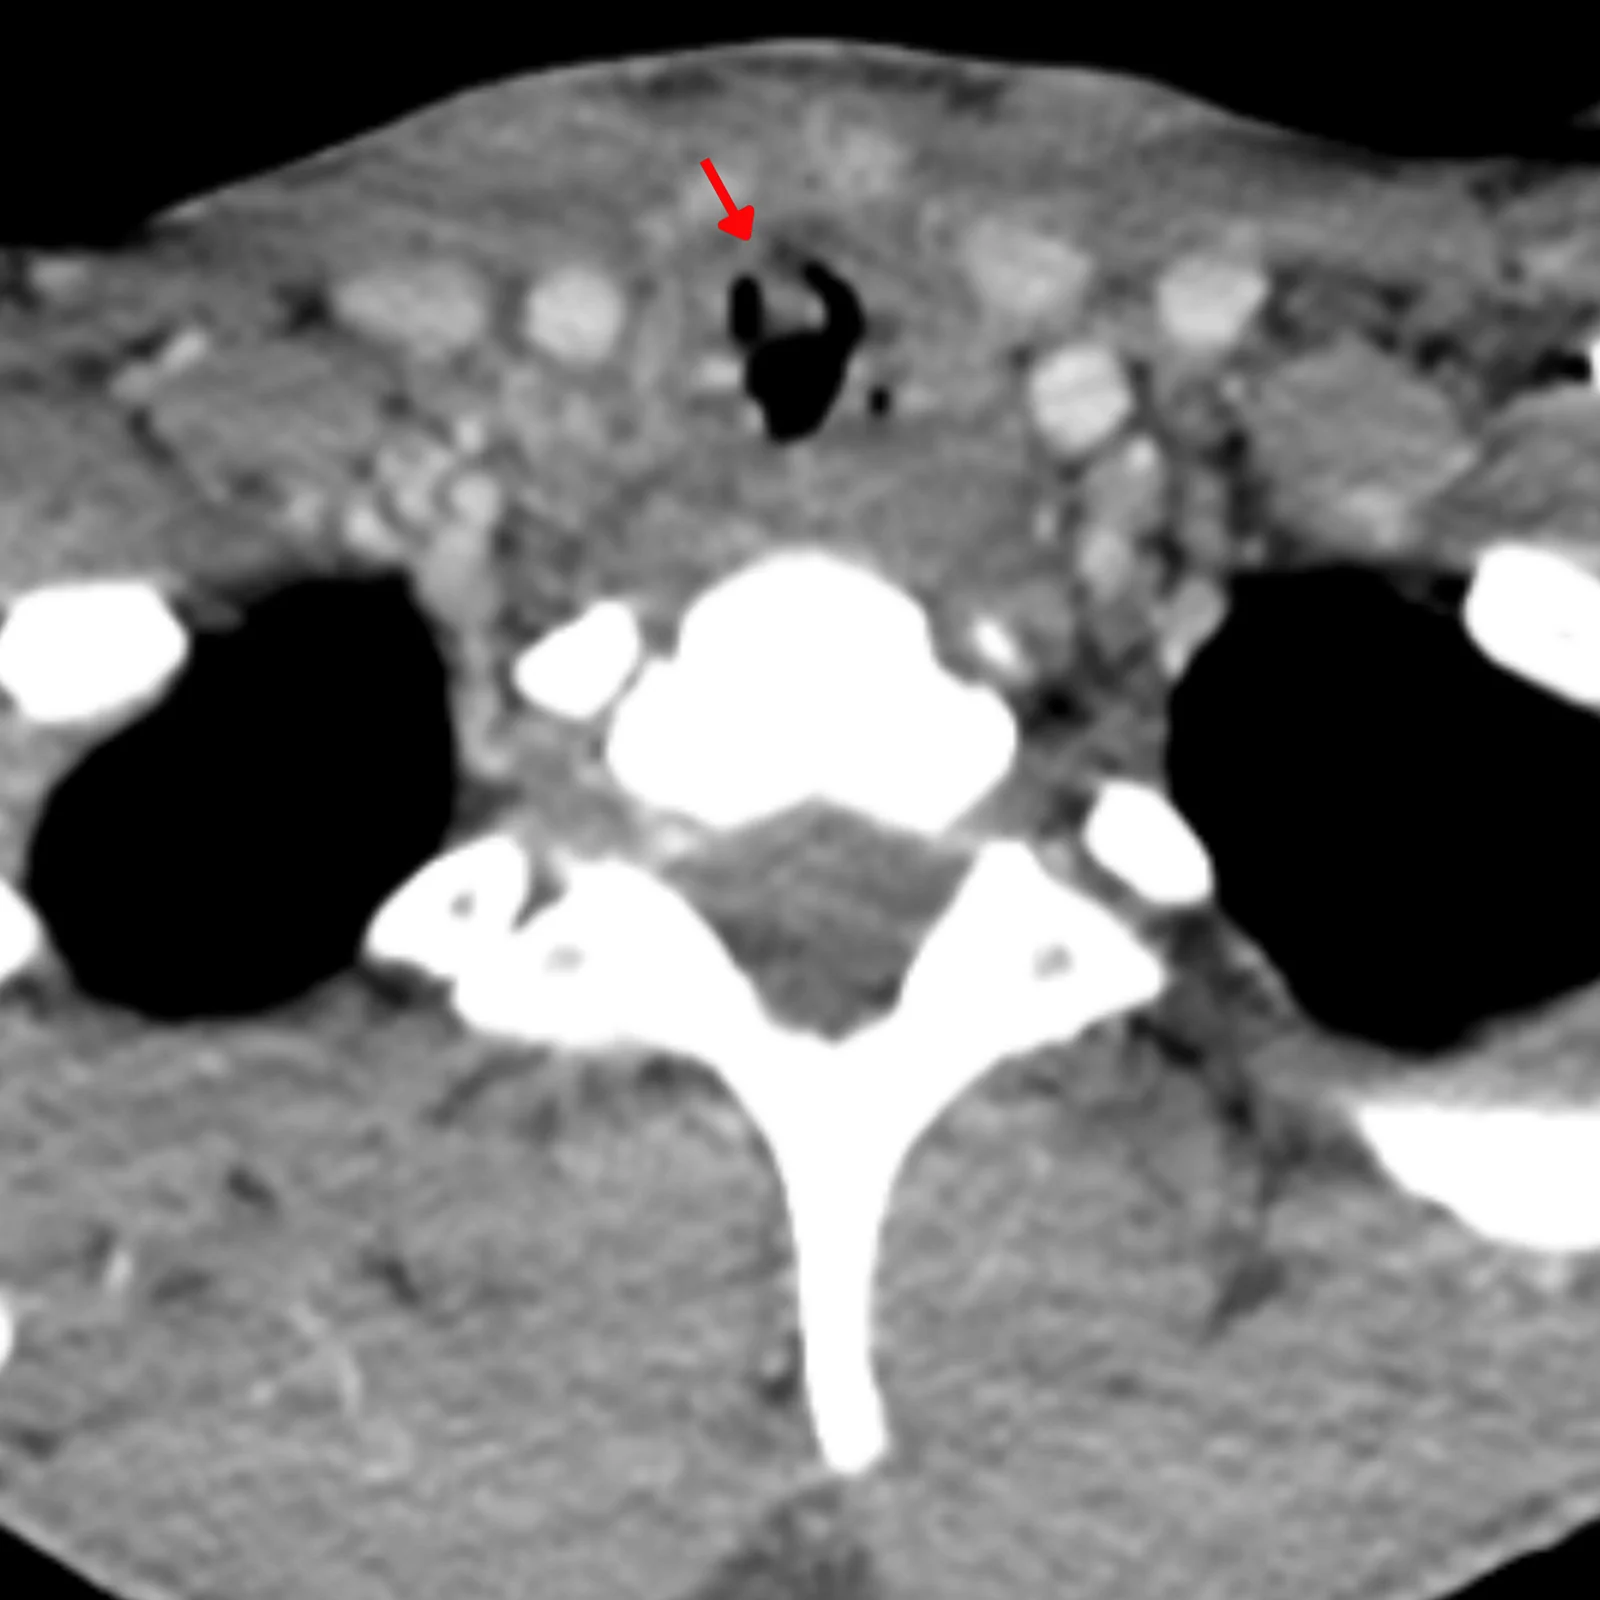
Contrast-enhanced CT scan of the neck showing a stenotic lesion located superior to the cricoid cartilage (red arrow)
